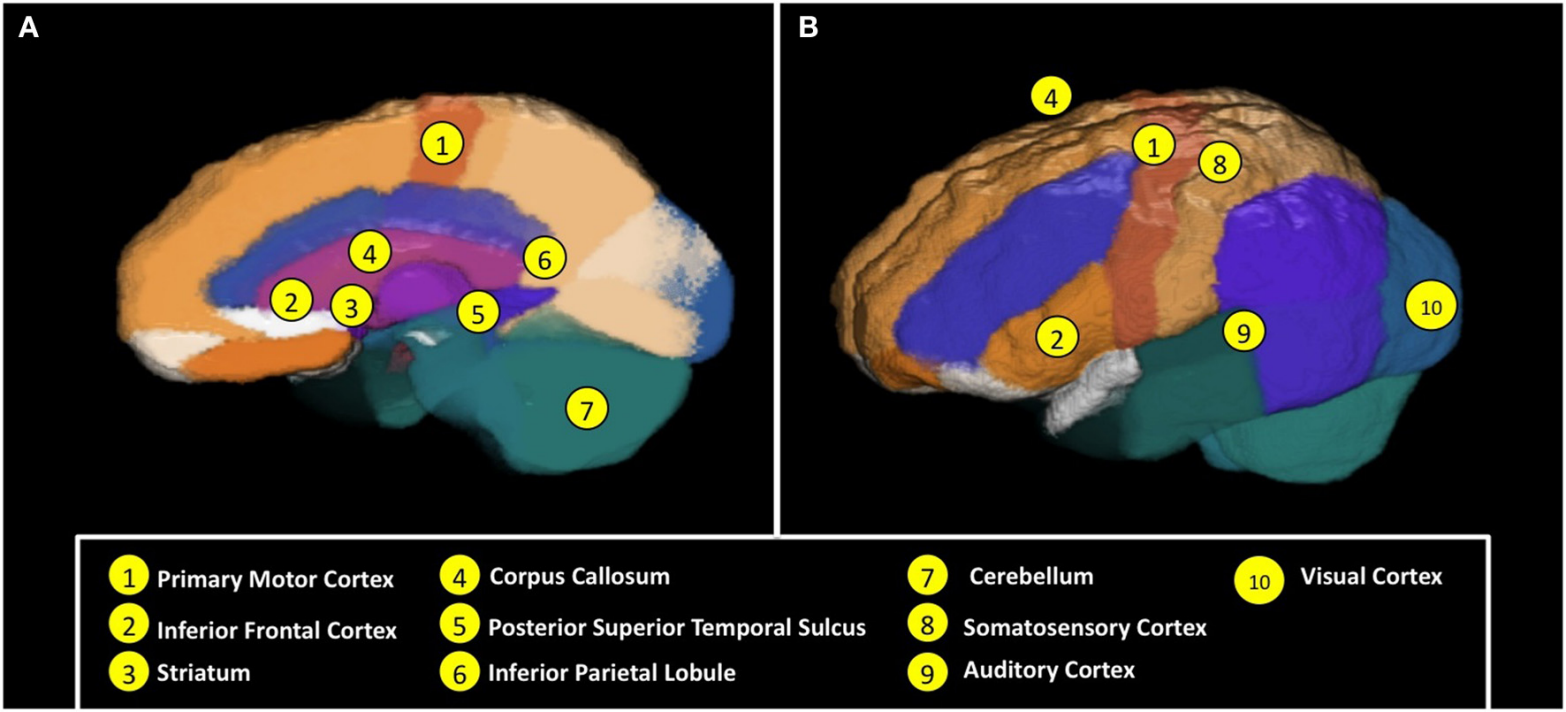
Neural regions and mechanisms involved in (A) motor functioning and action perception, and (B) neural coordination and connectivity for sensorimotor and speech/language functioning. See Table 1 for brief descriptions of these regions and associated mechanisms. Images of an average of 6-year-old child brain generated via the Magnetic Resonance Image database of Sanchez et al. (2012).
